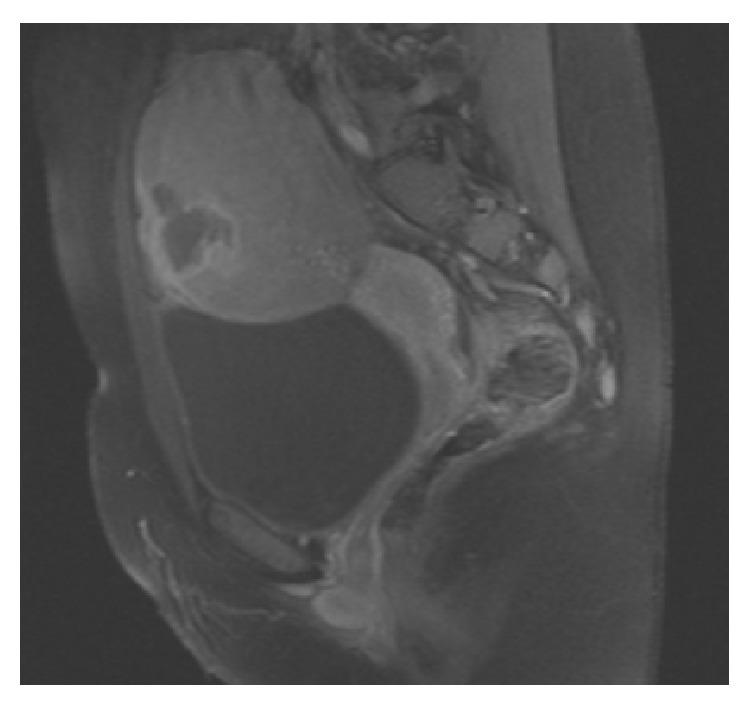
Sagittal T2-contrast-enhanced, fat saturated MR image of the mass.
